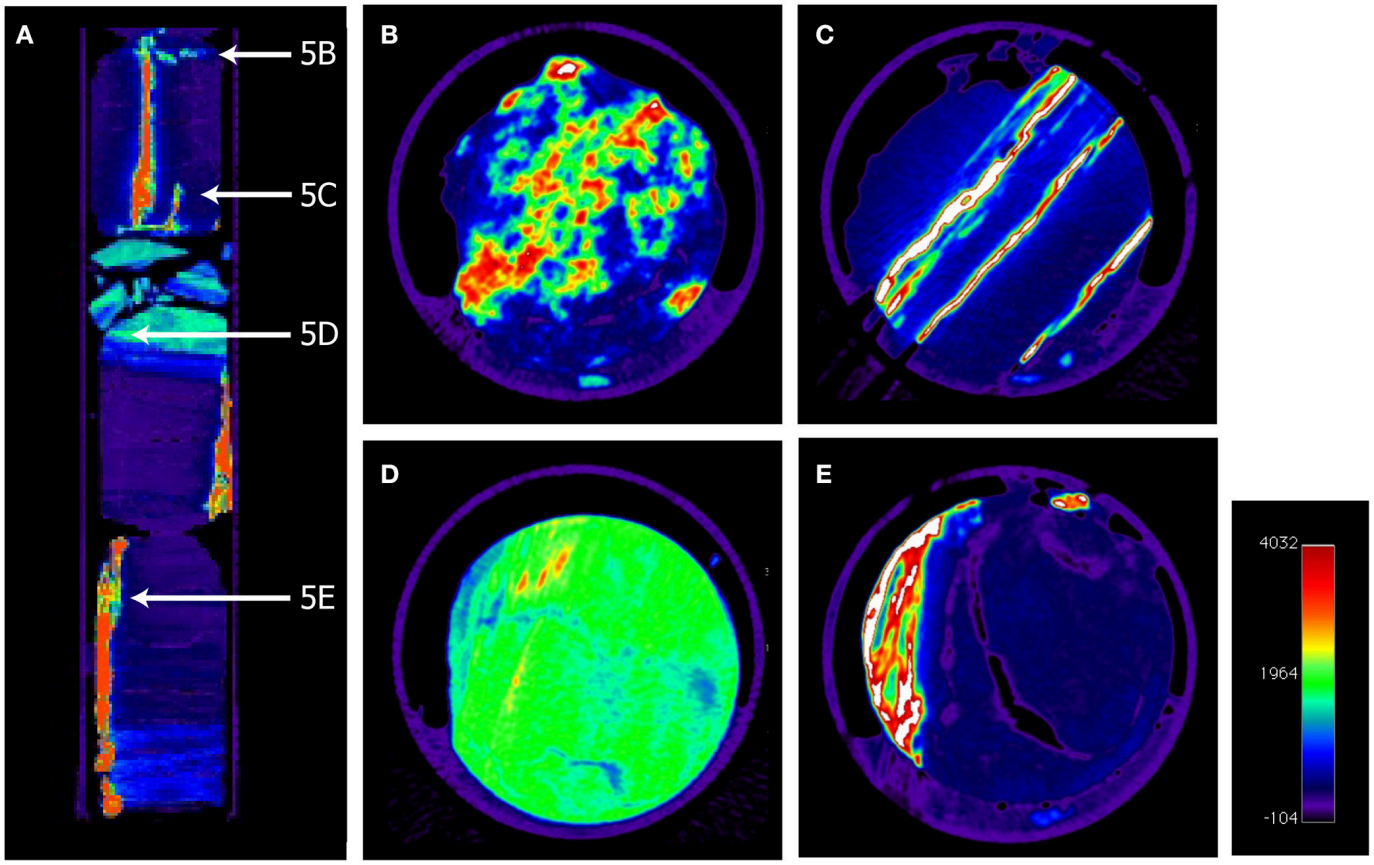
Colored x-ray computer tomography pictures of a 34 cm long core segment of core 30R-2 showing vertical pyrite veins and pyrite granules. (A) Vertical picture with indications of the positions of horizontal panels (B–E). (B) Patchy pyrite precipitates, (C) Multiple vertical pyrite veins, (D) Mud-coal interface with small pyrites, (E) multiple pyrite veins in coal cavities.
